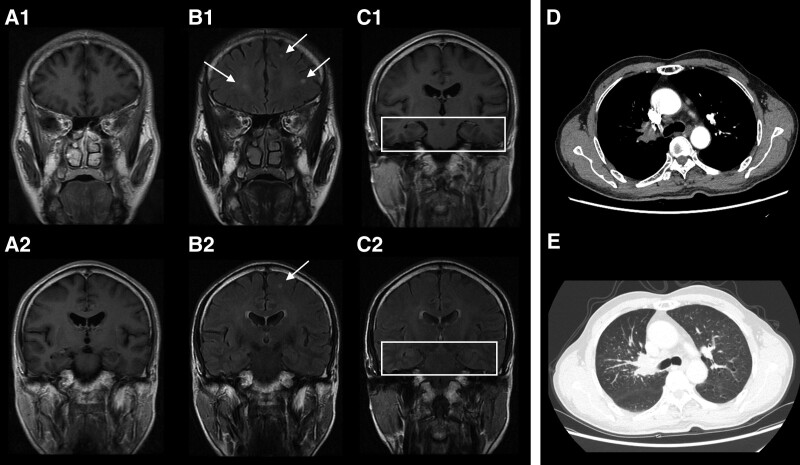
(A–B) T1W and T2W FLAIR imaging showed bilateral multifocal spotty and patchy signals in the subcortex and deep white matter of the bilateral frontal lobe, parietal lobe, and insula lobes. (C) No mesial temporal lobe abnormalities were seen in MRI. (D–E) Enlargement of hilum, amorphous soft tissue density shadow, and multiple small nodules and spot shadows in the right upper lobe of the lung; truncation and occlusion in the bronchus of the right upper lobe of the lung; and multiple enlarged lymph nodes in the mediastinum. FLAIR = fluid-attenuated inversion recovery, MRI = magnetic resonance imaging.
